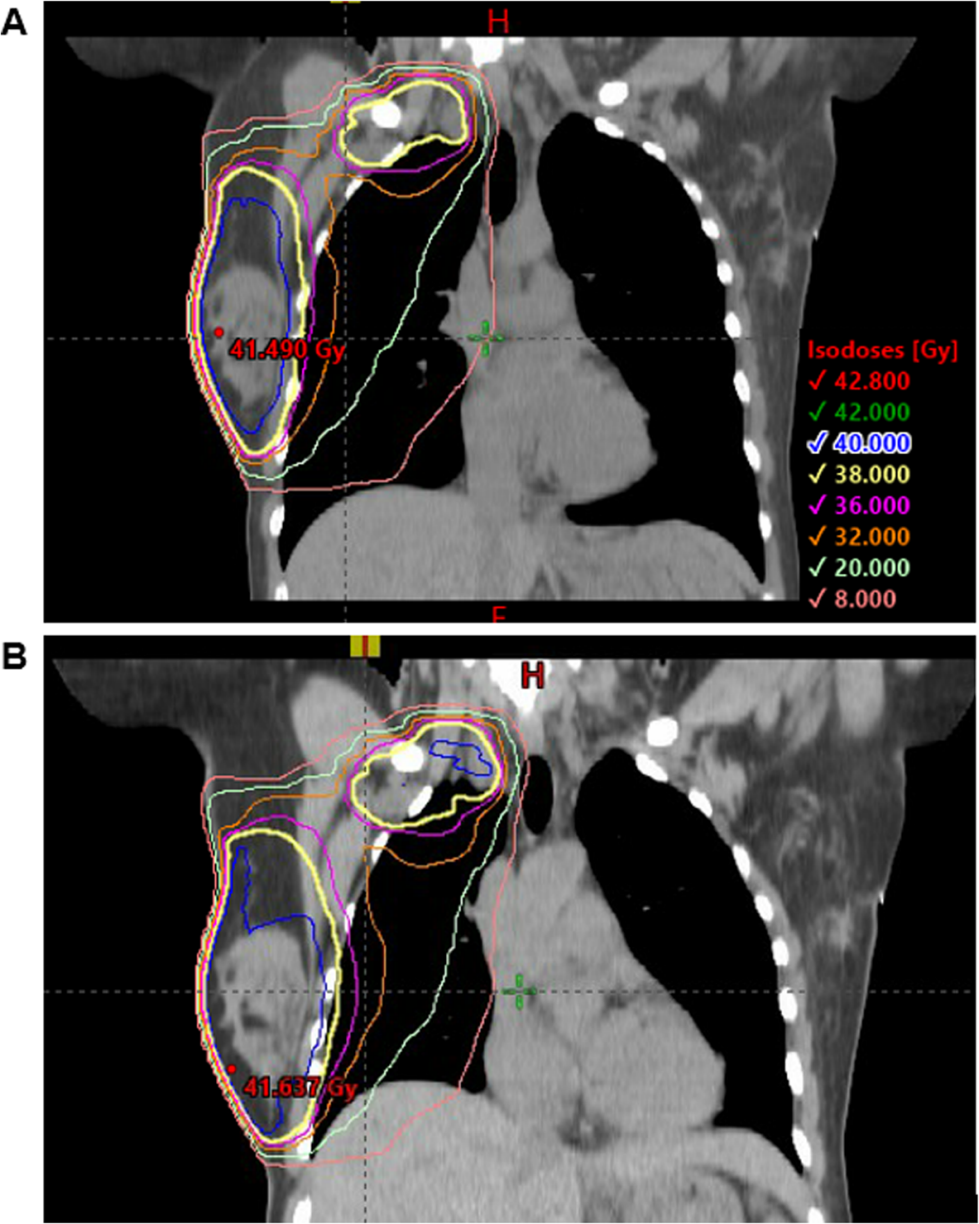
Coronal CT slices demonstrating the superior-inferior liver displacement between DIBH (a) and FB (b) for a breast plus RNI patient
